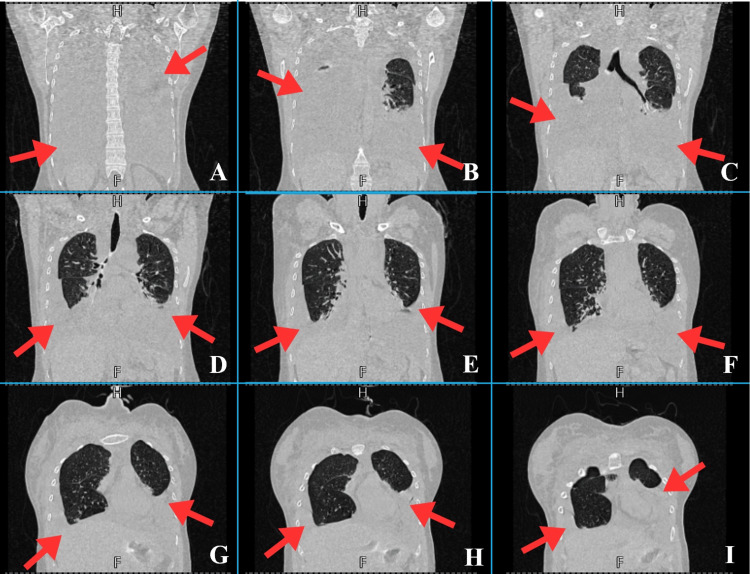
Sequential coronal high-resolution CT scans of the chest (panels A–I) exhibiting thoracic findings at three anatomical levels: posterior, middle, and anterior mediastinum (March 29, 2025). A and B: The posterior mediastinum shows a large bilateral pleural effusion (red arrows). C and D: The trachea and its bifurcation are visible, with moderate-to-large left-sided pleural effusion and moderate right-sided pleural effusion (red arrows), resulting in compression of the basal lung segments. E, F, and G: The middle mediastinum shows bilateral pleural effusions (red arrows) and increased pulmonary vascular markings, consistent with vascular congestion. H and I: The anterior mediastinum shows bilateral pleural effusion, resulting in passive atelectasis of the lower lobes of the lungs (red arrows).
